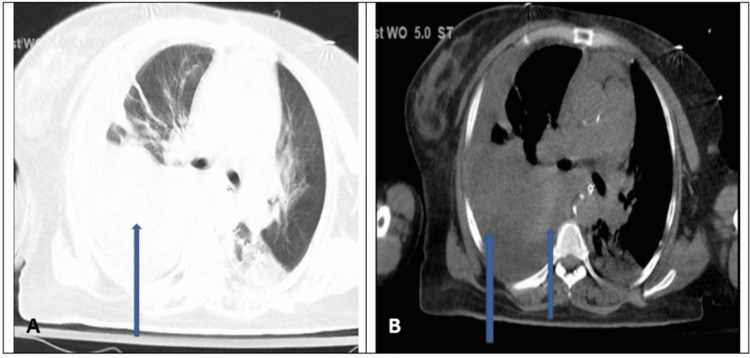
CT of the chest showing dense right lower lobe consolidation (blue arrow) (A) and large pleural effusion with a hyperintense lesion on the fluid (blue arrow) (B), suggestive of early septa formation. CT: computed tomography
